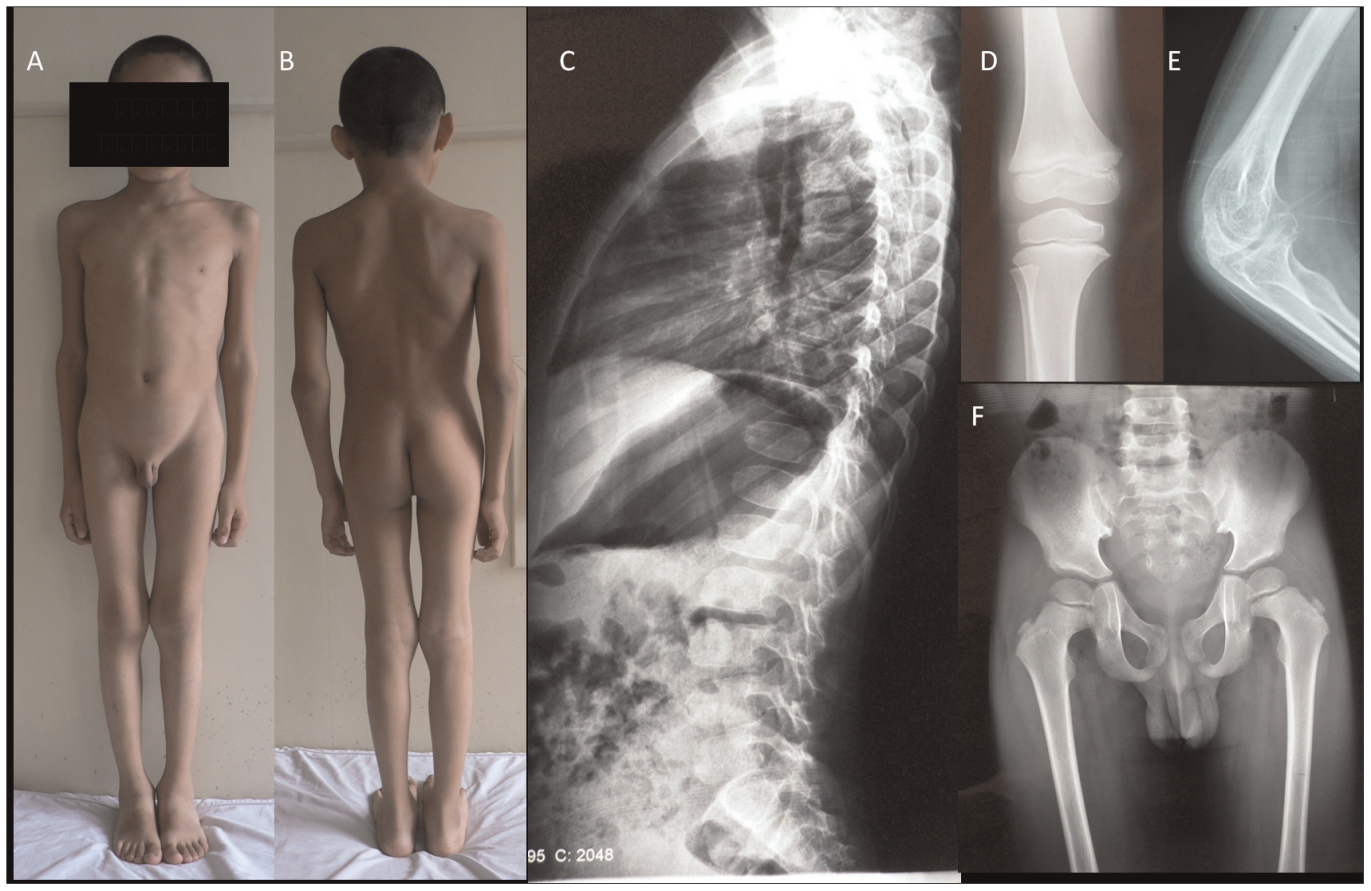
The photographs and X-ray images of the proband in family 1. (A&B) Short-trunk disproportionate dwarfism and enlargement of the elbow and genual joints; (C) platyspondyly and ovoid anterior end-plate of vertebral bodies; (D) widened tibial metaphysis; (E) widened cubital metaphysis; (F) a normal pelvis at 5 years of age.
